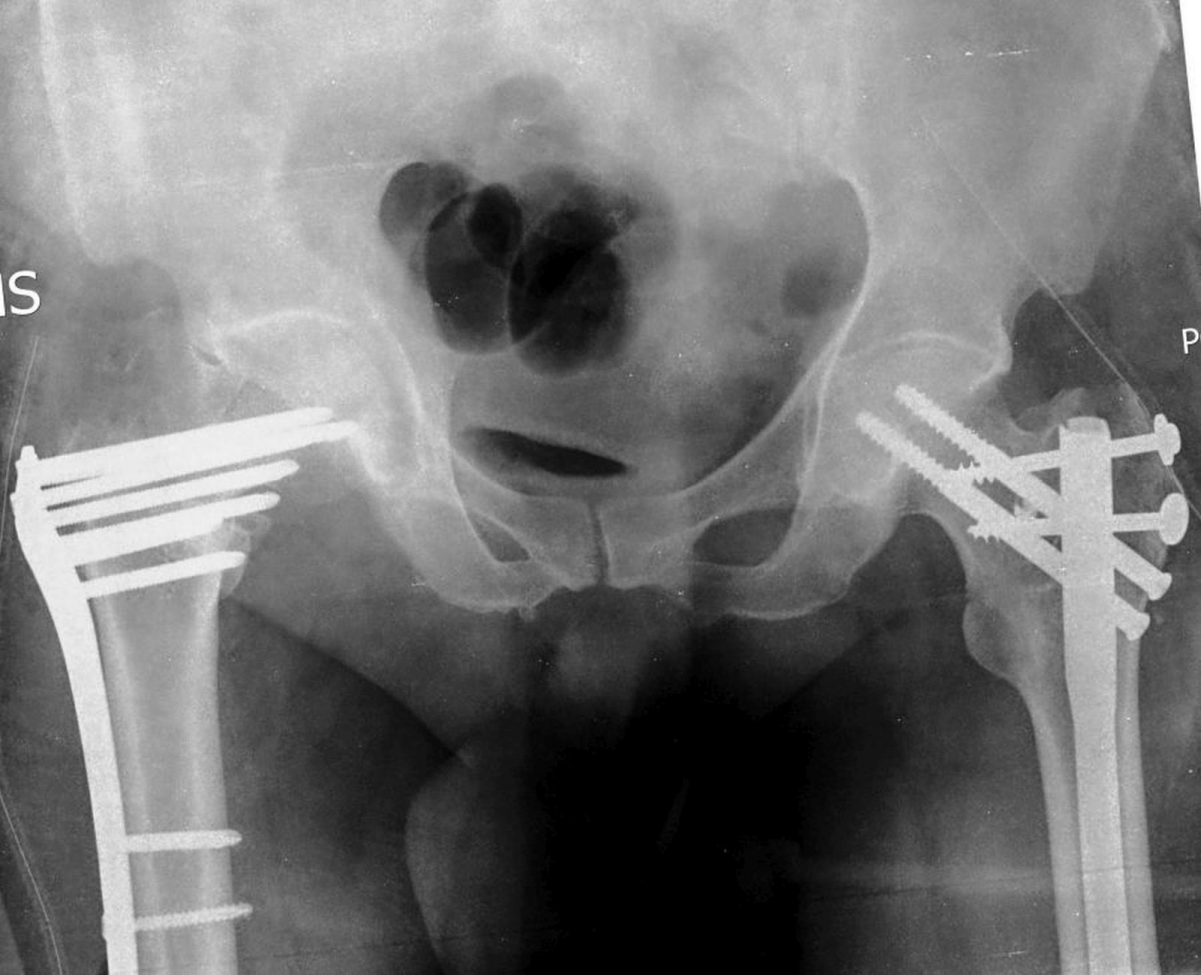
Six months post-op x-ray of the pelvis showing signs of healing of both the trochanteric fractures
